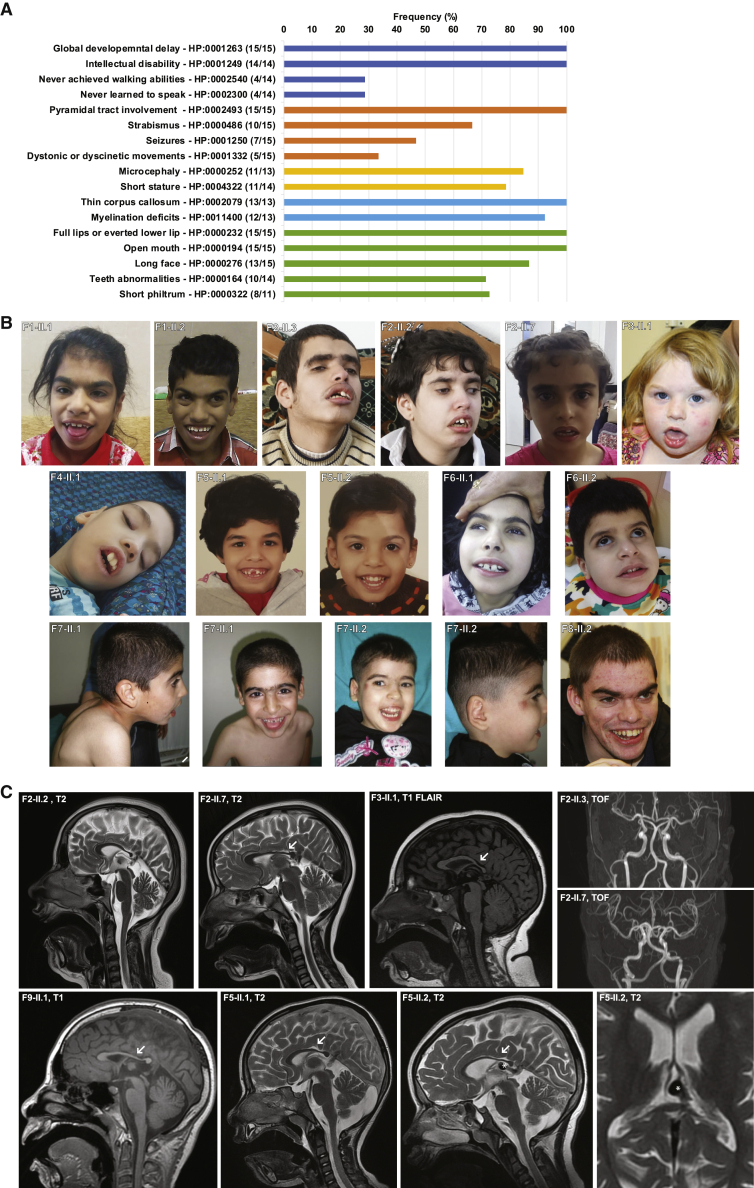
Phenotypic features of probands with bi-allelic *BCAS3* variants (A) Prevalence of signs and symptoms in probands with bi-allelic *BCAS3* variants. The numerator and denominator in brackets indicate the number of affected probands and the number of probands assessed for the respective feature, respectively. (B) Facial photographs of 14 affected individuals with mild dysmorphic facial features, including full lips with an everted lower lip; short philtrum; mispositioned, wide-spaced large teeth; and synophrys. Furthermore, ptosis is present in F1-II.1, F2-II.2, and F2-II.3, and strabismus can be seen in several photographs except for in those of F4-II.1, F5-II.1, F5-II.2, F7-II.1, F7-II.2, and F8-II.2. (C) Exemplary MRI of probands from families F2, F3, F5, and F9. Midsagittal T2/T1 showing a thin dysgenic corpus callosum (white arrow), especially of the posterior part (splenium). This is present in all available MRIs. Coronal TOF-MRA displaying normally developed large arterial blood vessels of the brain. A vascular malformation, most likely a vein of Galen malformation, was identified in one proband (F5-II.2) and is marked with a white asterisk on the T2 images.
